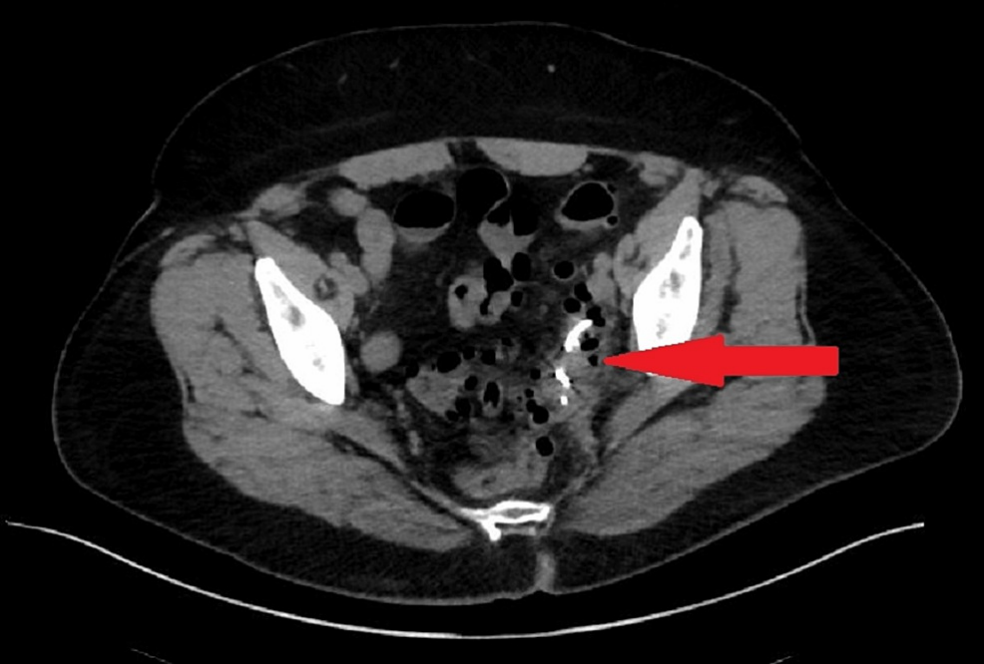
Computed tomography scan with the foreign body in the sigmoid colon with surrounding inflammation
